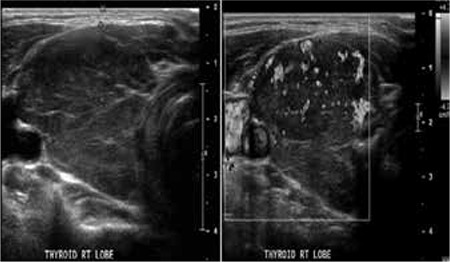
Thyroid ultrasound demonstrated diffusely hypo echoic, asymmetrically enlarged thyroid gland with diffusely increased vascularity, but with no discrete focal mass lesions (only right thyroid lobe shown).
